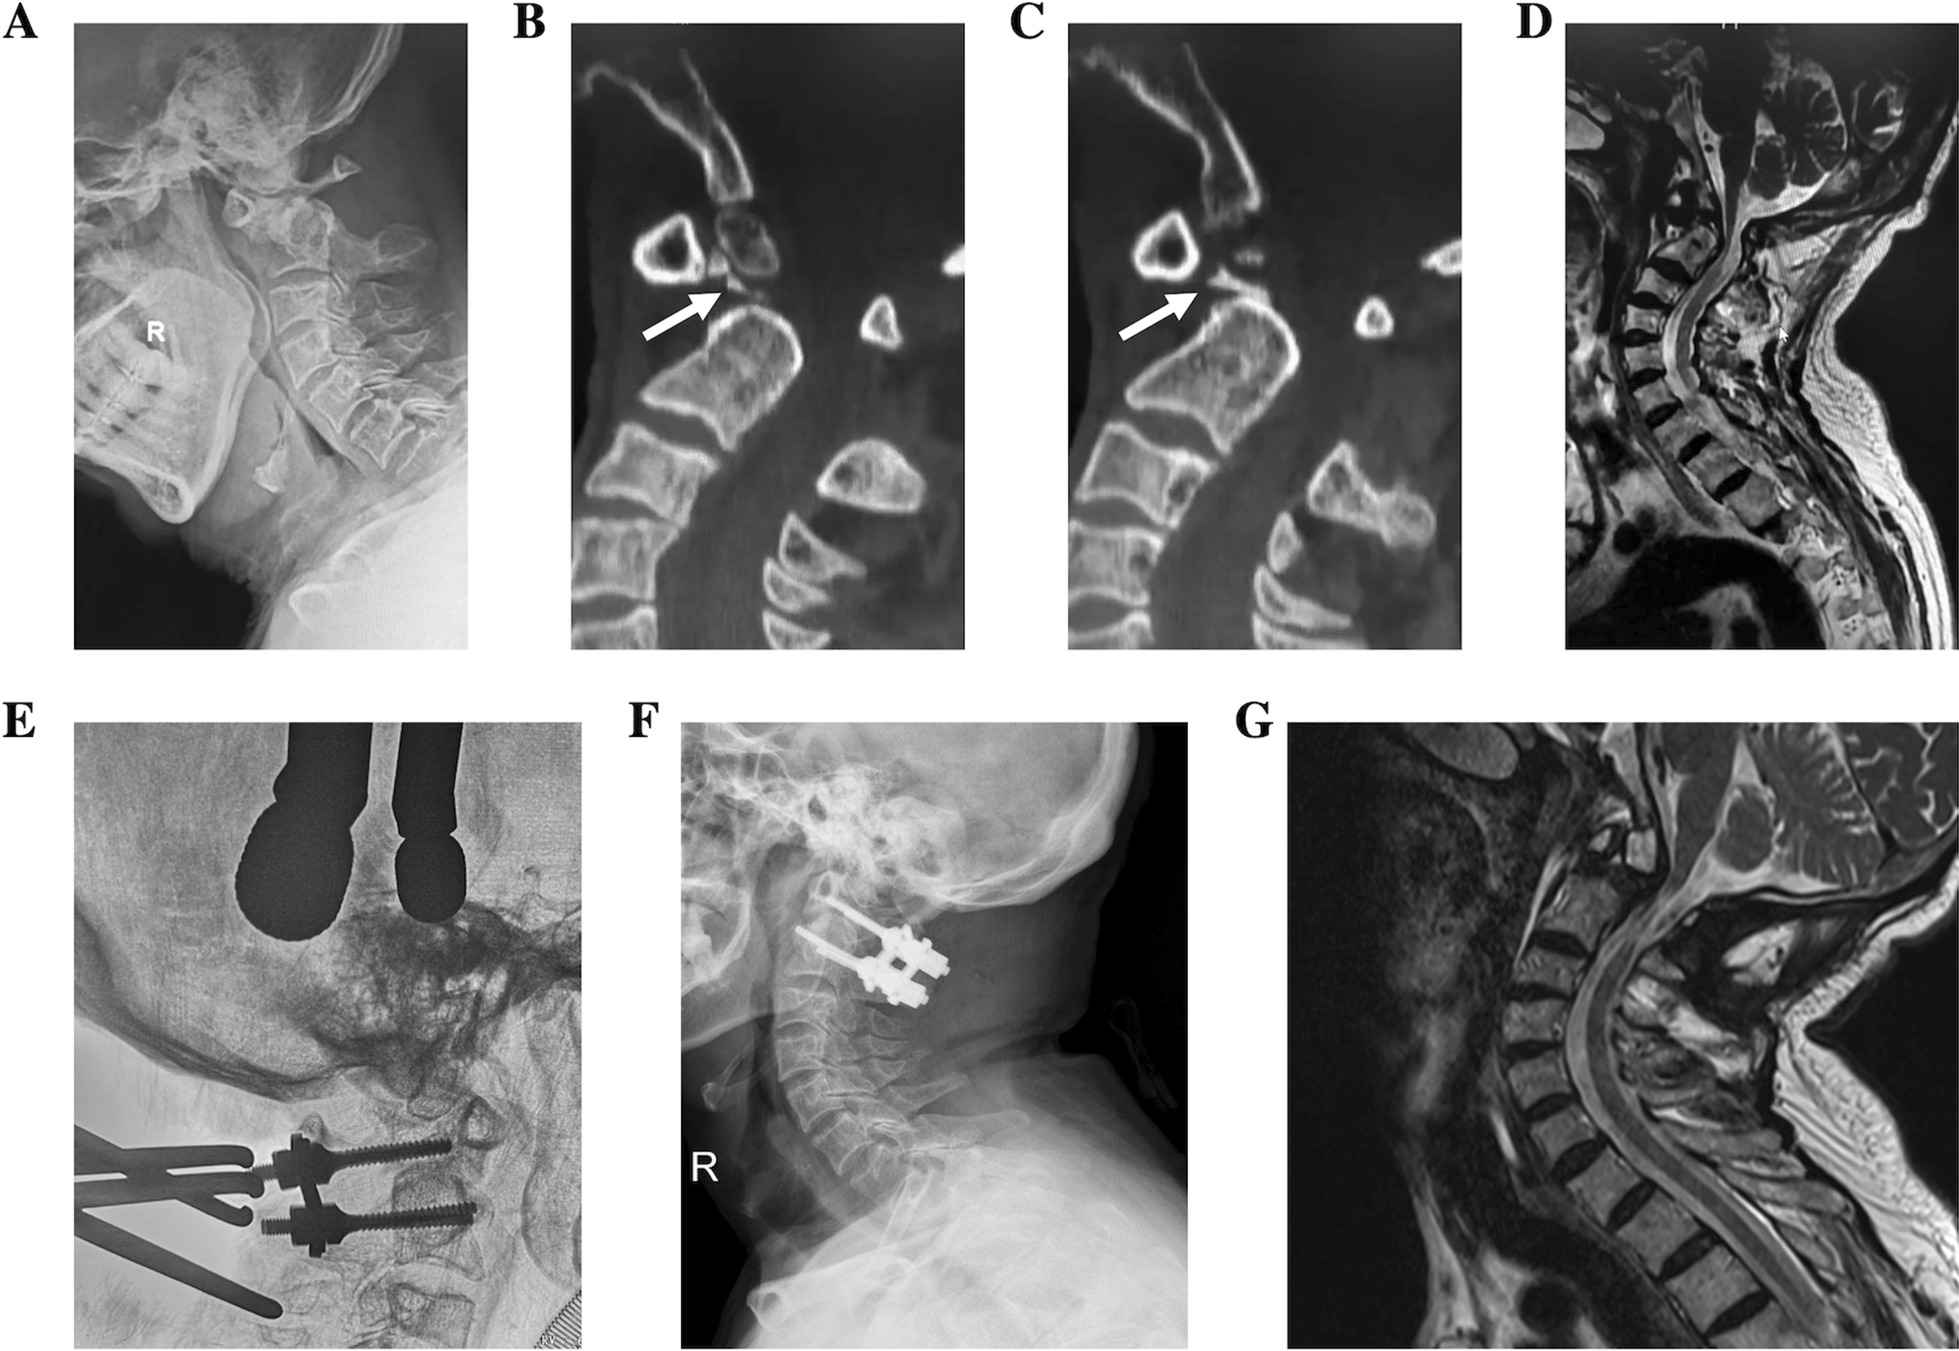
A 56-year-old male patient was admitted for numbness of the right limbs with walking instability for 11 months. Preoperative lateral X-ray showed OO with AAD (a), and some discontinuous bony structures were seen between the anterior arches of the atlas and the axis in computed tomography (CT) sagittal reconstruction (b, c, white arrow). Magnetic resonance imaging (MRI) showed compression of cervicomedullary junction (CMJ) (d). Reduction of the atlantoaxial dislocation by intraoperative high-weight skull traction failed, and reduction less than 50% was classified as type III. Following transoral release and posterior fixation and fusion, satisfactory reduction were achieved (e, f), and MRI showed the elimination of CMJ compression postoperatively (g)
